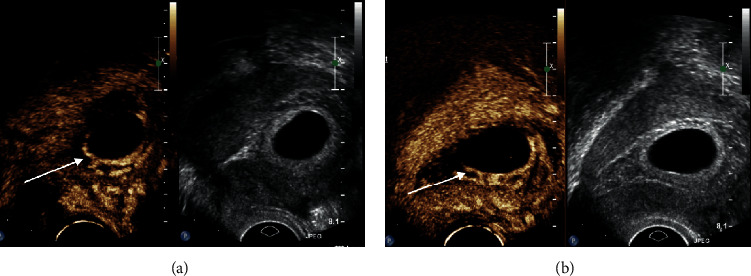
Findings from transvaginal contrast-enhanced ultrasonography before and after mifepristone administration in patients with intrauterine pregnancy. (a) Before mifepristone treatment. (b) After mifepristone treatment.
